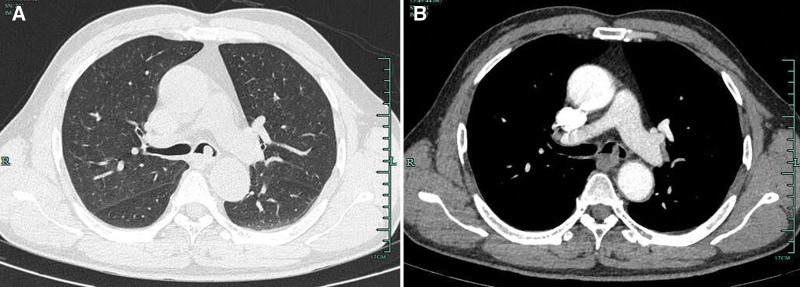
Chest CT finding of the left main bronchus nodule. (A) Lung window chest CT scanning image showed a 10 × 8 mm round, smooth-surface nodule located in left upper main bronchus. (B) Enhanced mediastinal windows show the nodule was heterogeneous contrast enhanced. CT = computed tomography.
